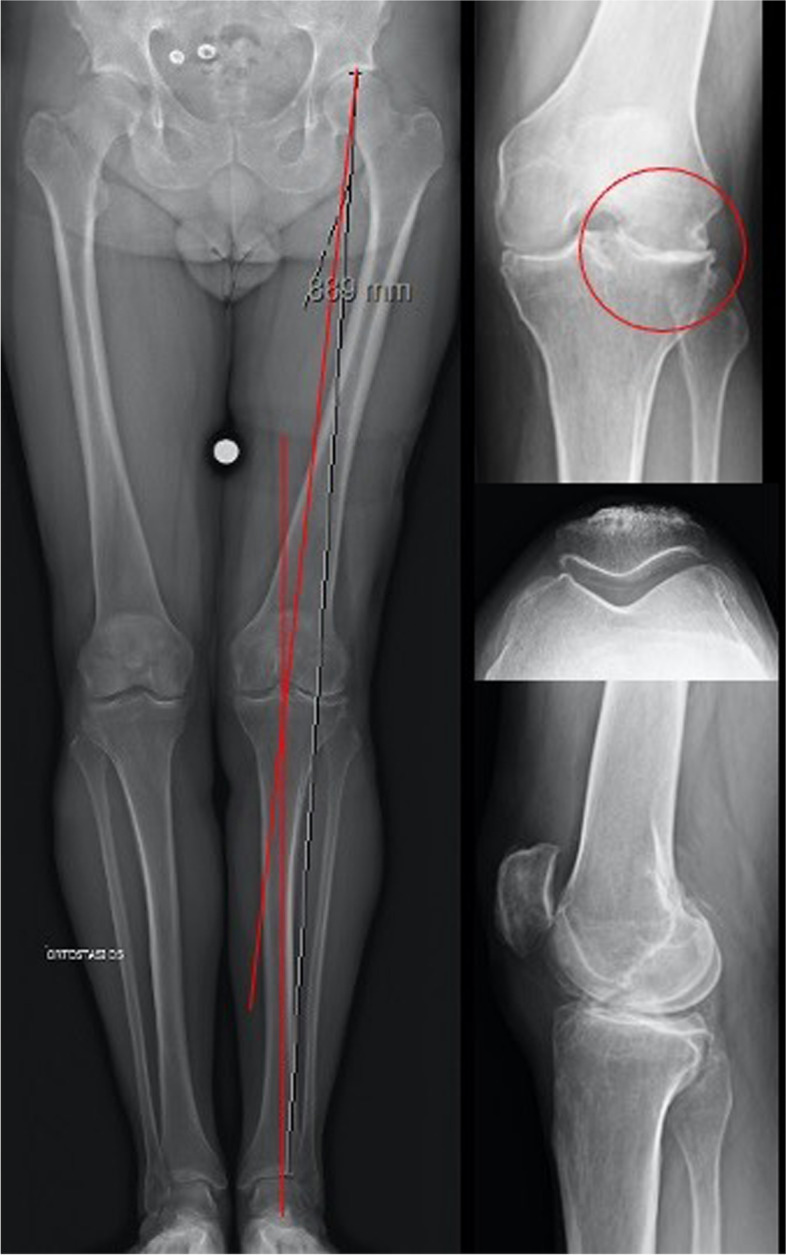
Preoperative X-rays evaluation of a 56 years-old male with a native varus morphotype, who underwent a lateral open meniscectomy 15 years before in his left knee. Consequently, he developed a grade IV lateral OA, a grade III medial OA and a valgus alignment
